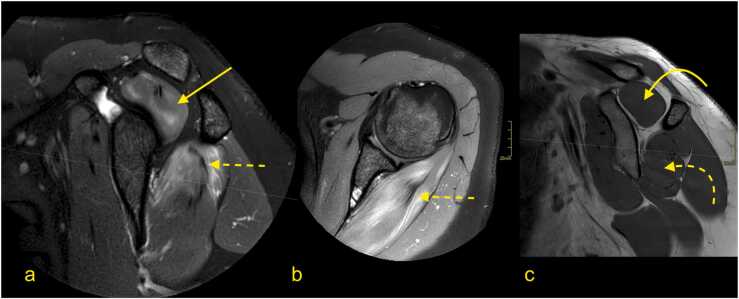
Parsonage-Turner syndrome. A 31-year-old patient with pain in the left shoulder. (a) T2-weighted TSE MRI with fat suppression, (b) PD-weighted MRI with fat saturation, and (c) T1-weighted TSE MRI. There was diffuse muscle edema in the supraspinatus (arrow) and infraspinatus (dashed arrow) muscles without atrophy (curved arrow and curved dashed arrow). This represents the involvement of the suprascapular nerve; however, no lesion was demonstrated in the infraclavicular part of the BP.
